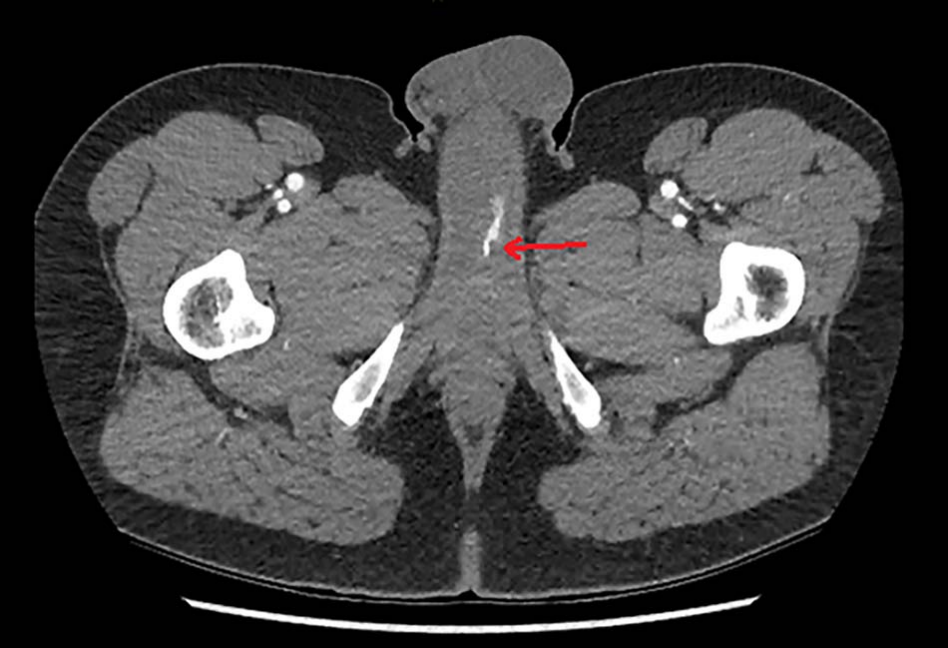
CTA demonstrating an axial view of contrast enhancement within the region of the left cavernosal artery, indicative of an arterial pseudoaneurysm (red arrow).
